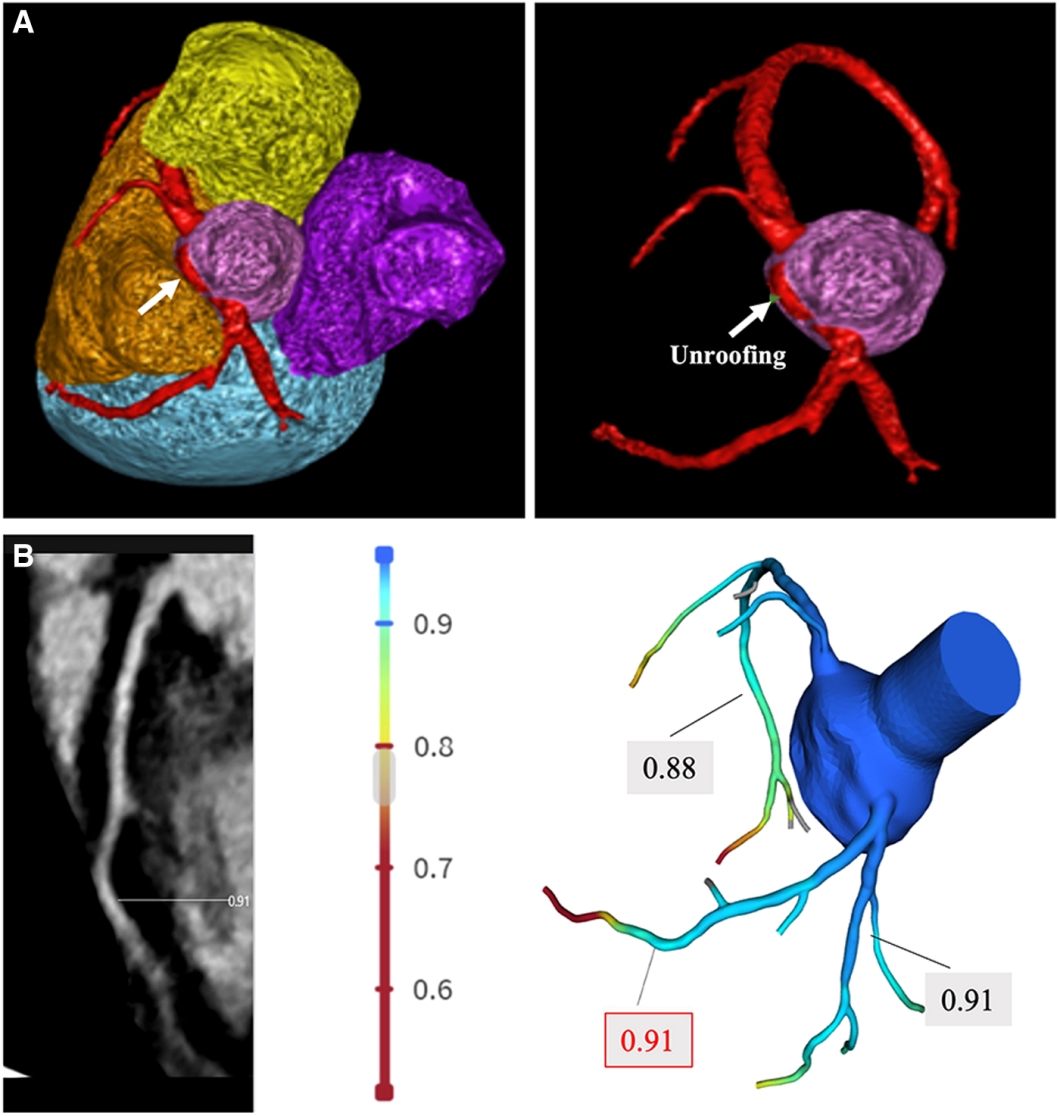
Post-procedure CT-FFR measurement. (A) Coronary CTA shows revascularization of the LCA. The intramural segment of the coronary artery of the LCA is unroofed (white arrow) and corrected to originate from the left sinus of Valsalva. (B) CT-FFR technique was performed 1 week after the revascularization procedure, and the result showed a significantly improved FFR of the LCA (0.91) and normal range FFR of the LCX (0.91) and RCA (0.88).
